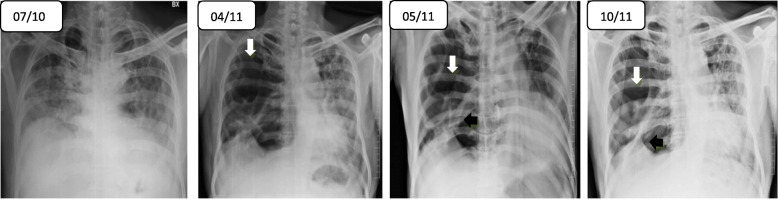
a–d Consecutive chest x-ray PA view shows progressive increase in lucencies in the right hemithorax (white arrow) with fibrotic changes (black arrow) and mediastinal shift towards the left
